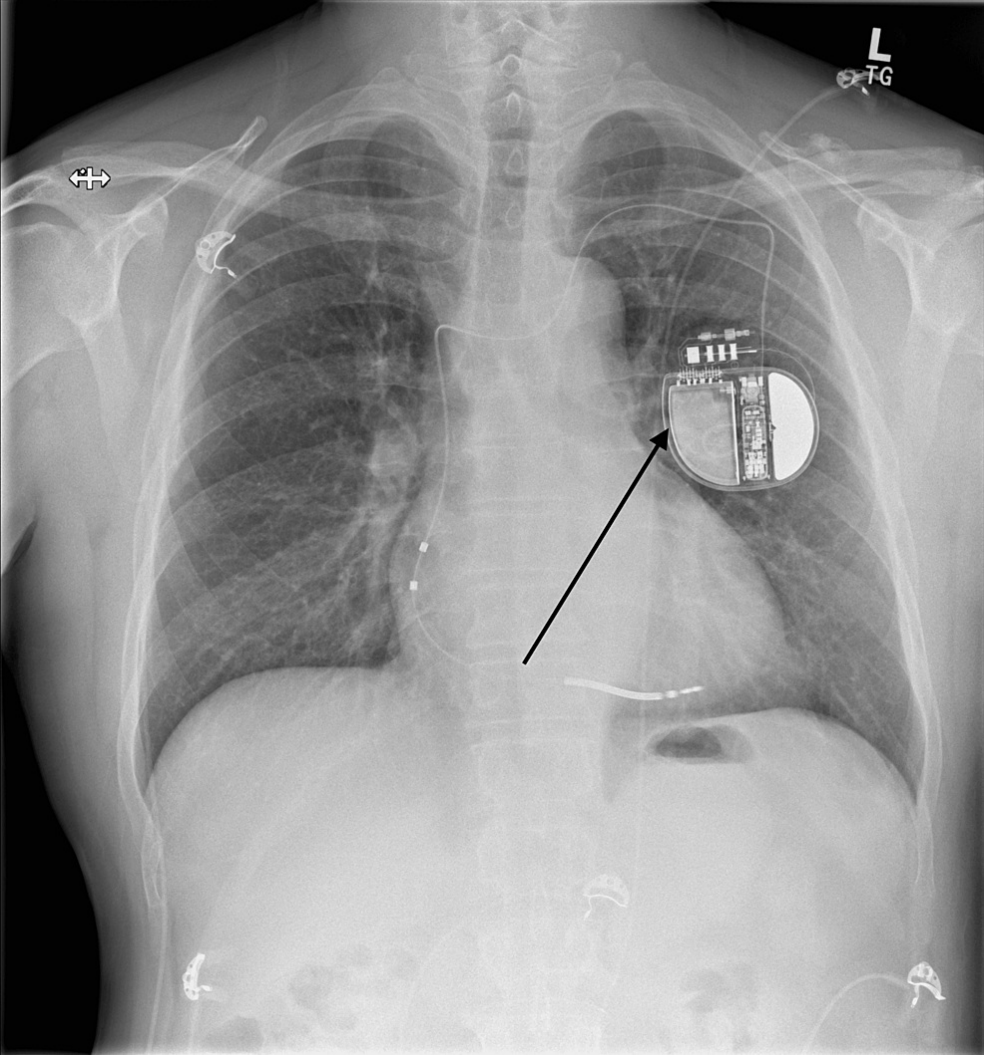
Chest X-ray post dual chamber implantable cardioverter defibrillator (ICD) implantation (arrows showing ICD on left side of chest)
